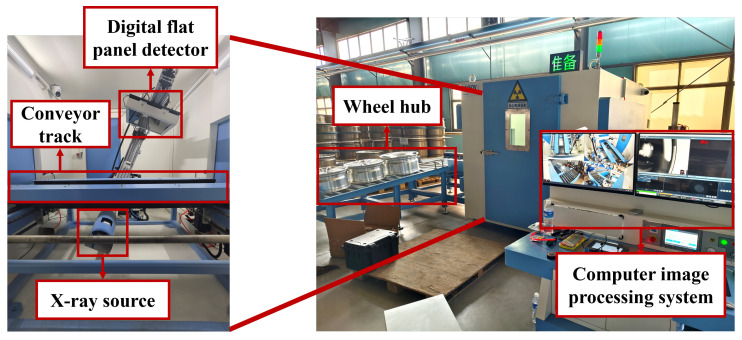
X-ray imaging data acquisition device.
